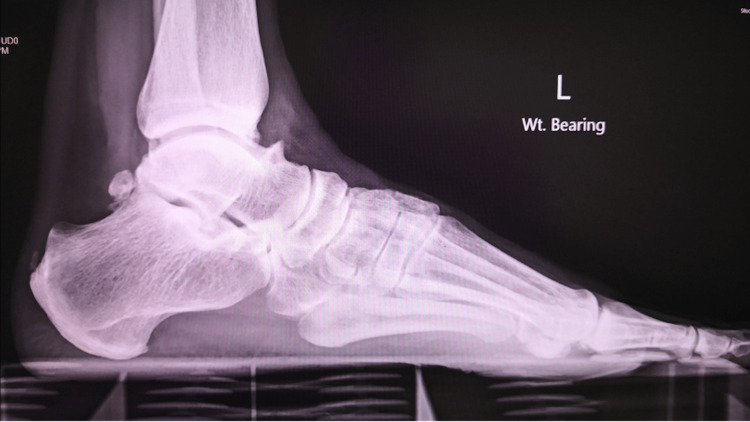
Pre-operative weight-bearing radiographs of the patient’s left foot.
